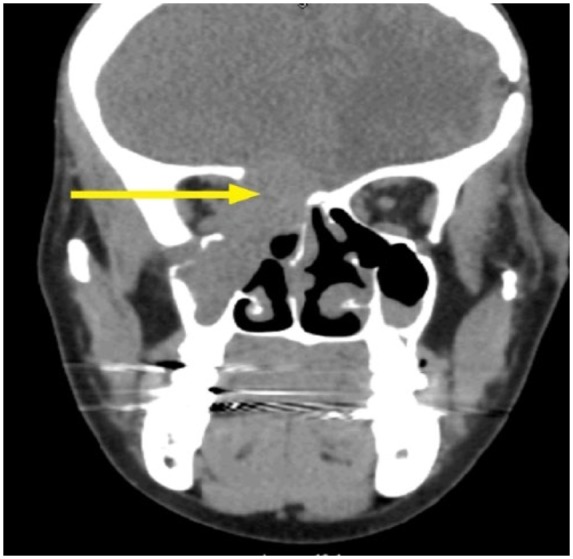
Computed tomography scan of the brain and maxillary sinus mass with bony erosion of the cribriform plate.
